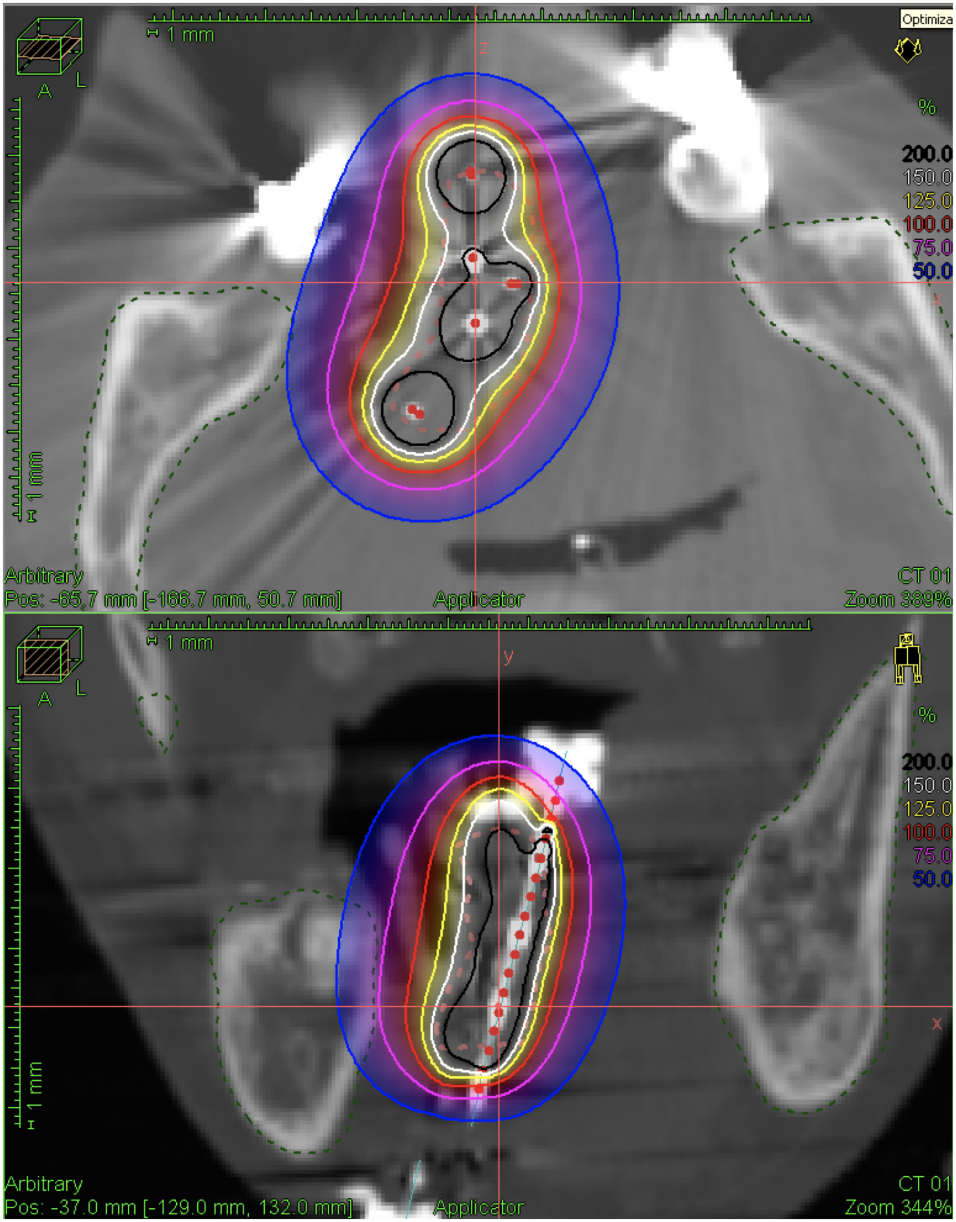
Modular spacer during 3D-CT planning without lead-shield (i.e. eliciting no artifacts).
